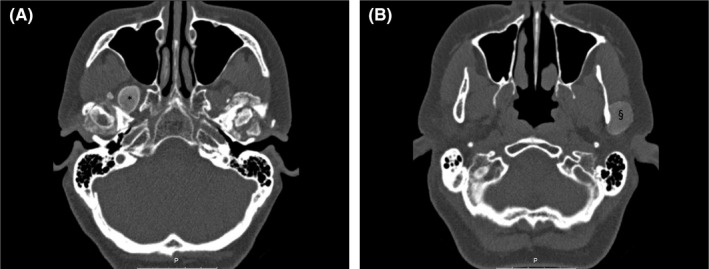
A, Axial CT scan showing extensive calcification with deformation and arthrosis in both temporomandibular joints and surrounding structures including a well‐circumscribed calcified nodule in the right pterygopalatine fossa region (*). B, Axial CT scan showing well‐defined calcified nodule (§) in the left preauricular region caudal to the temporomandibular joint
